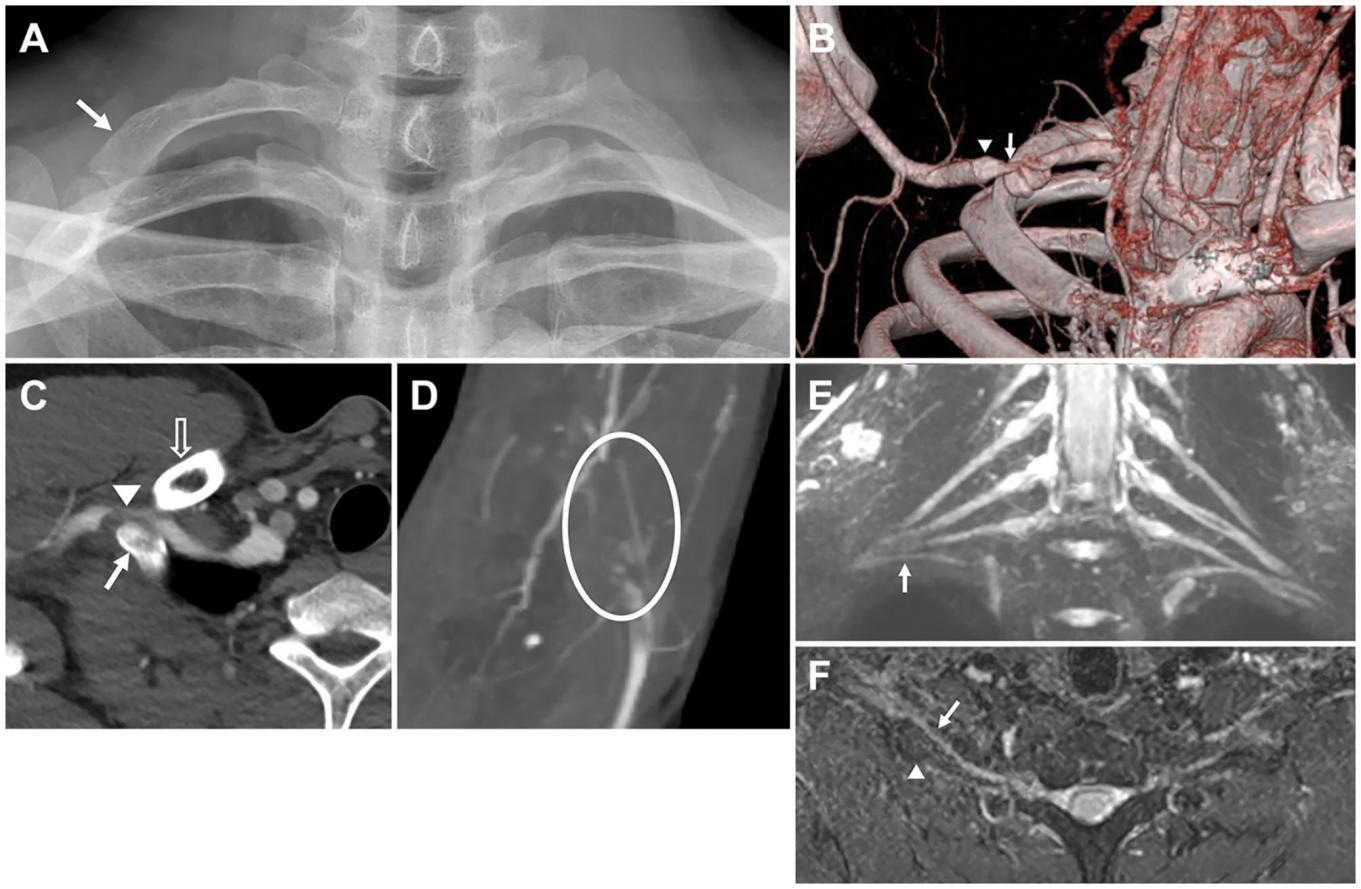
Imaging findings for Patient 2. (B–D) Computed tomography (CT) angiography performed with 180° shoulder abduction. (E,F) Brachial plexus magnetic resonance imaging (MRI). (A) Plain radiograph of the cervical spine showing upwardly convex curvature and an anomaly of the right first rib (arrow). (B) When viewed from the front, a reconstruction image using volume rendering shows stenosis (arrow) and post-stenotic dilatation (arrowhead) of the right subclavian artery. (C) Axial image showing the spatial relationships of the anomalous right first rib (arrow) articulating with the second rib, narrowed subclavian artery (arrowhead), and clavicle (voided arrow). (D) Maximum intensity projection (MIP) image showing thromboembolic occlusion of the right brachial artery at the elbow level (circle). (E) Coronal T2-weighted fat suppression MIP image showing a high-riding right T1 extraforaminal root and lower trunk (arrow) compared to those on the opposite side. Abnormal signal intensity of the brachial plexus was not definite. (F) Axial T2-weighted fat suppression MIP image showing anterior angulation of the right lower trunk (arrow) by the anomalous right first rib (arrowhead).
